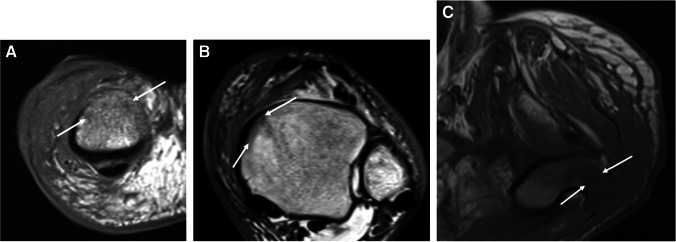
Patterns of T1 signal alteration. Short-axis T1-weighted image of the first proximal phalanx shows a hazy, reticular pattern of T1 marrow signal abnormality, where patchy areas of fat signal are seen amidst a background of reticular low T1 signal (arrows, A), while an axial T1 image of the ankle shows a subcortical distribution of signal abnormality, with a thin linear region of low T1 signal adjacent to the medial tibial cortex (arrows, B). An axial image of the hip shows T1 features compatible with osteomyelitis, with a confluent pattern and medullary distribution of marrow signal abnormality (arrows, C), involving a geographic area of the medullary canal along the greater trochanter
